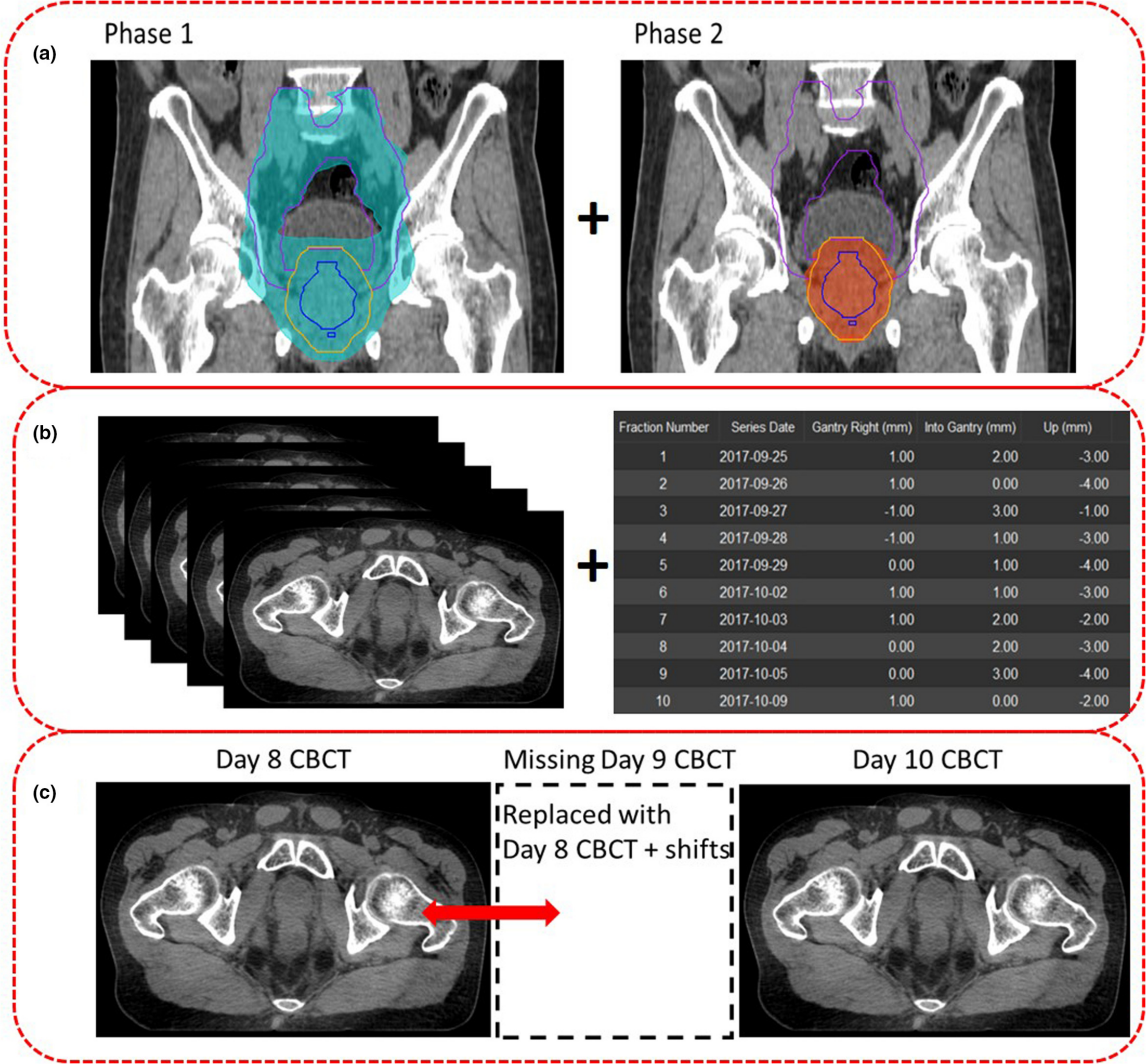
Pre‐processing steps prior to performing dose accumulation. (A) RT plan information from Eclipse TPS, (B) Daily CBCT images and treatment couch shifts information, (C) CBCT data management.
